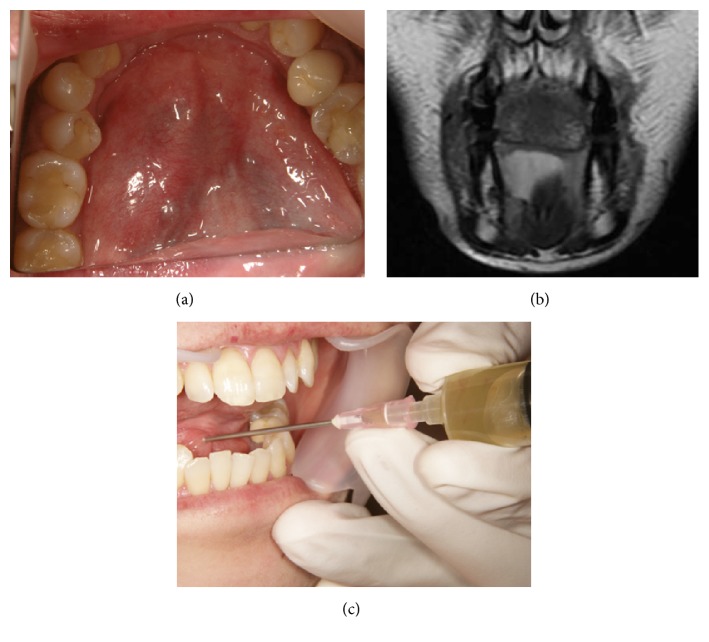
Appearance of the ranula at the first medical examination shows swelling of the anterior floor of the mouth (using mirror) (a). T2-weighted magnetic resonance images before treatment, showing the high-intensity areas in the right sublingual regions (b). The aspirated content from the cystic lesion was yellowish and mucinous (c).
